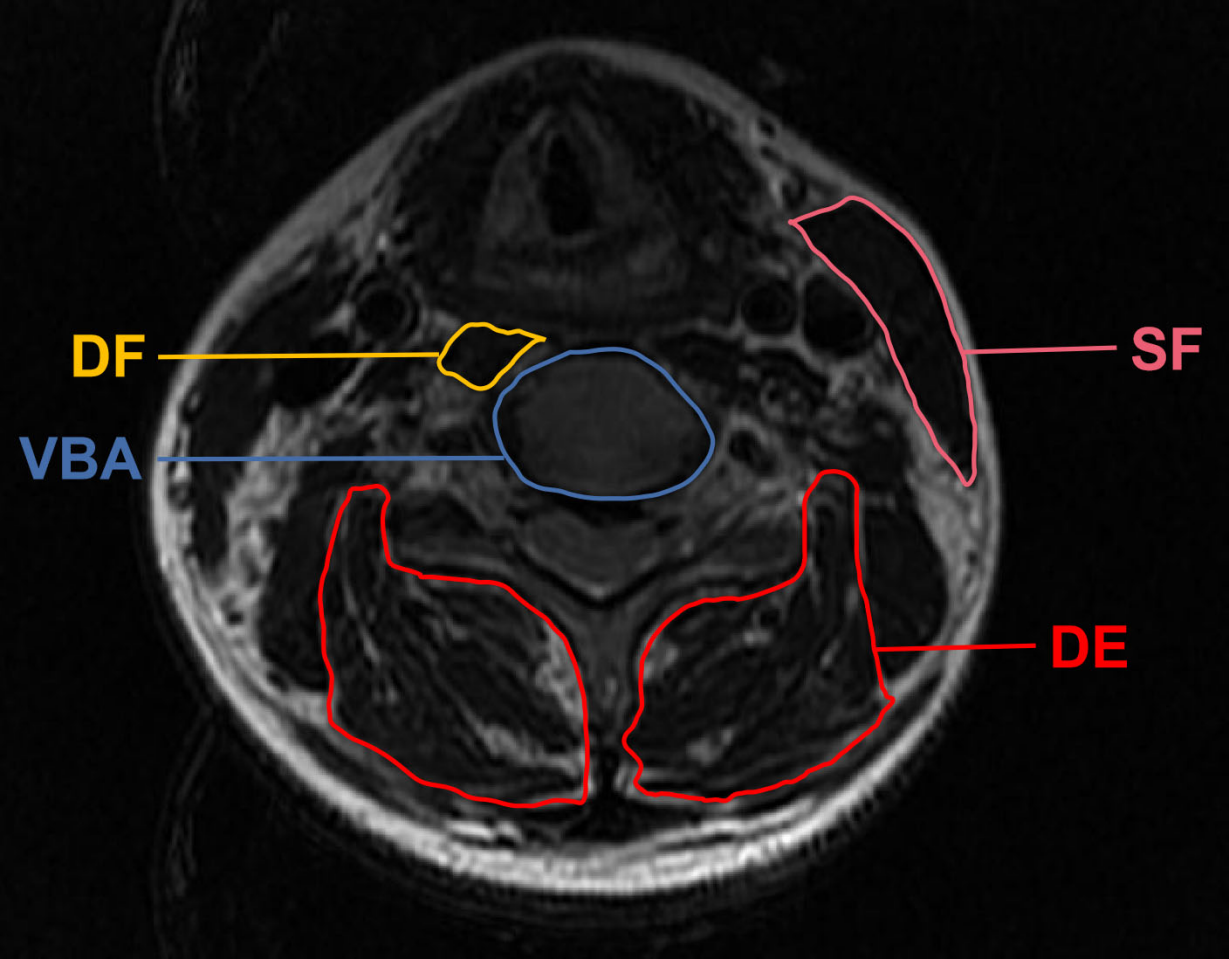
Demonstrative axial T2 magnetic resonance imaging sections of the regions of interest applied in measuring the cross-sectional area. SF, superficial flexor; DF, deep flexor; DE, deep extensor; VBA, vertebral body area.
